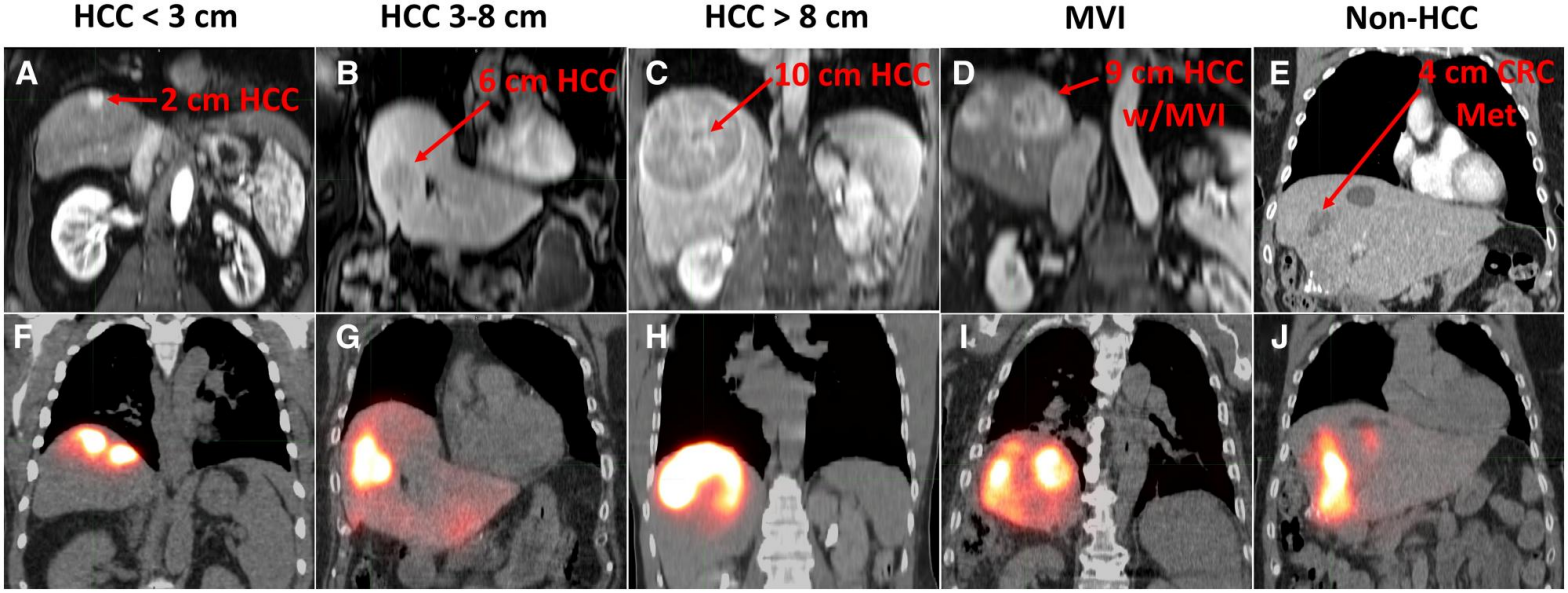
Morphological (A-E) and MAA-SPECT/CT (F-J) imaging for the example cases in each of the 5 tumor categories analyzed in this study. The target lesion and its size are identified in each morphological image. Full clinical parameter details for these 5 cases are included in Table 2 for reference. HCC = hepatocellular carcinoma; MAA = macroaggregated albumin; MVI = macrovascular invasion; CRC Met = colorectal cancer metastasis.
